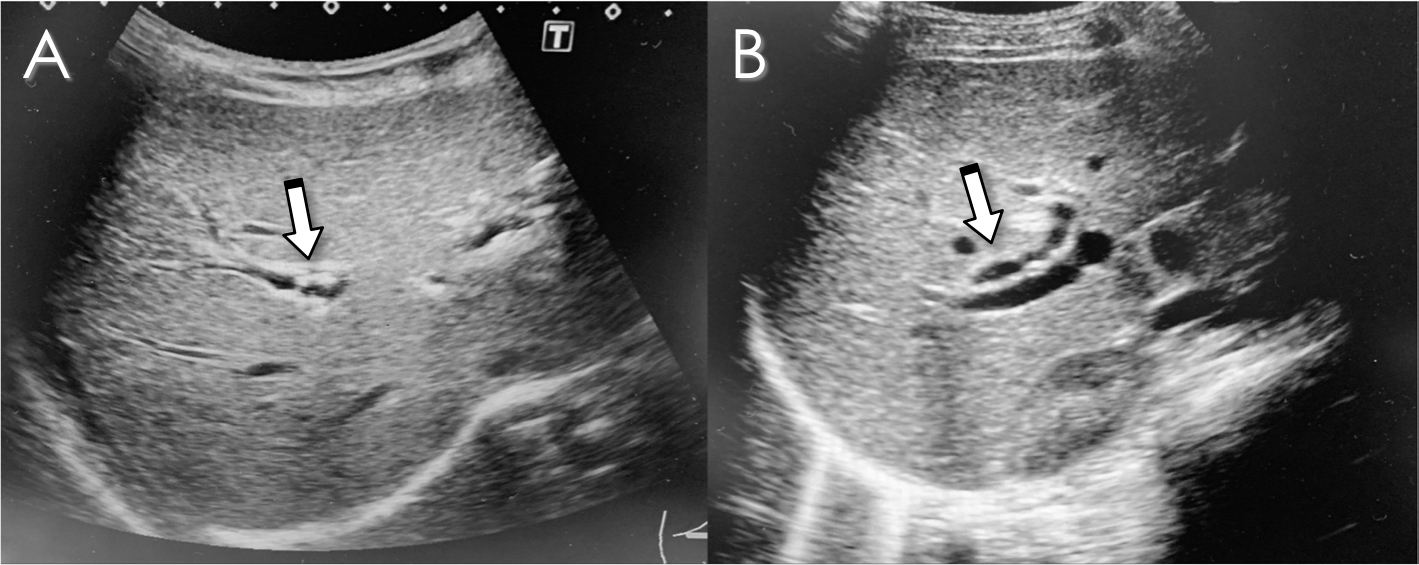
Ultrasound findings in a case in which an IHBD stone disappeared with oral UDCA. A Before treatment. Arrow indicates an IHBD stone in the right hepatic duct. B After treatment. Arrow shows the absence of the stone
